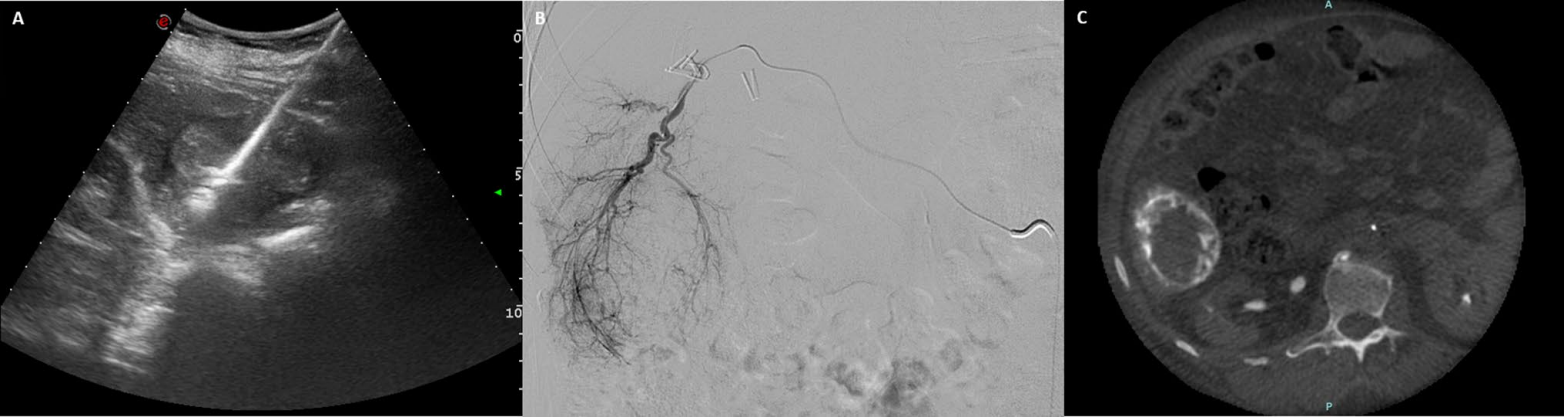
a–c Combined TA-TACE treatment. a. Microwave ablation of a 55-mm HCC nodule of the VI liver segment performed using a 14G probe (maximum power output 150 W, ablation time 3 min and 30 s), b Digital Subtraction Angiography (DSA) performed 24h after TA, showing the ablation zone surrounded by an hyper-vascular rim, c unenhanced cone beam CT performed at the end of chemoembolization, demonstrating the complete peripheral coverage of the ablation zone
